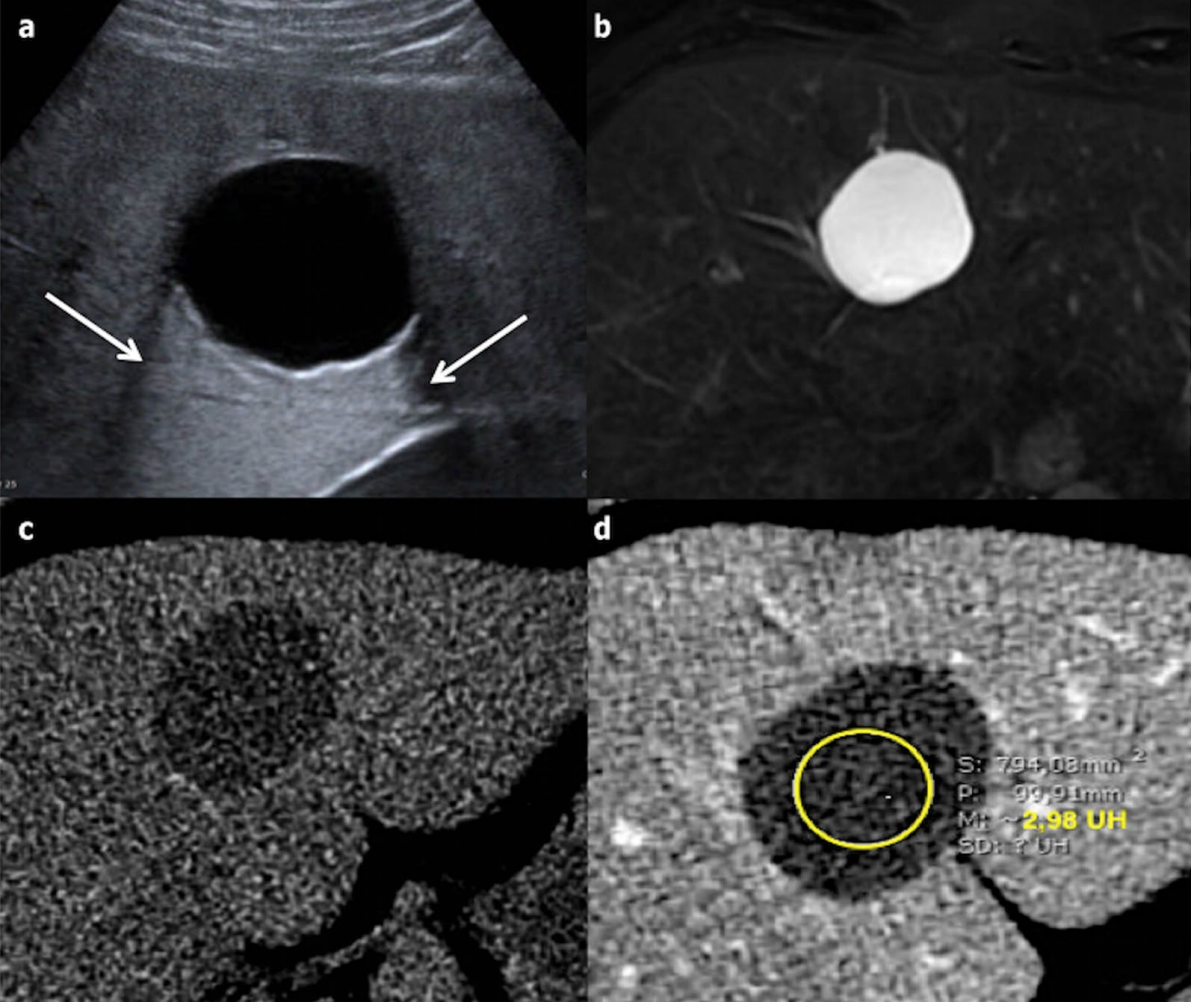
Incidentally found simple hepatic cyst in an asymptomatic 64-year-old male. a Ultrasonography shows a round homogeneous anechoic cystic hepatic lesion, well-circumscribed and without any mural nodule or vegetation. The arrows show an increased through transmission, confirming the cystic nature of the lesion. b T2-weighted magnetic resonance imaging shows a highly hyperintense round lesion. c and d Computed tomography without and with contrast on portal venous phase shows a round homogeneous non-enhancing hypoattenuating lesion (3 Hounsfield Units)
